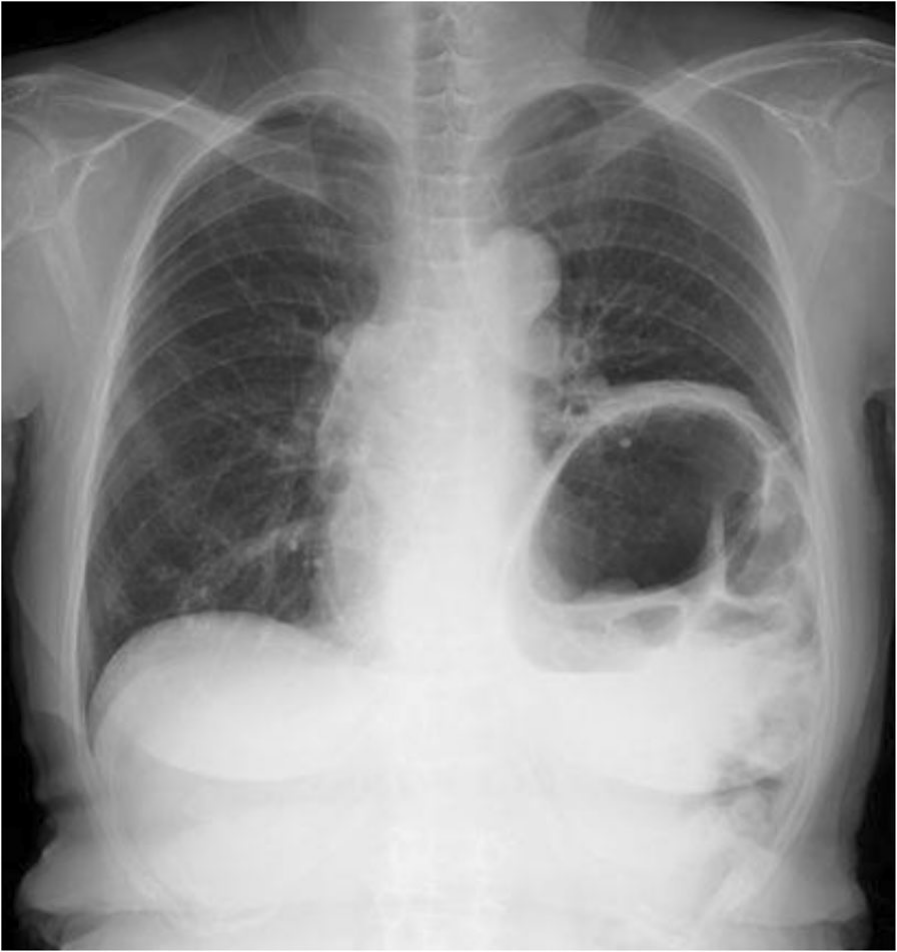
Chest X-ray before diaphragm plication. The left diaphragm was elevated before plication
